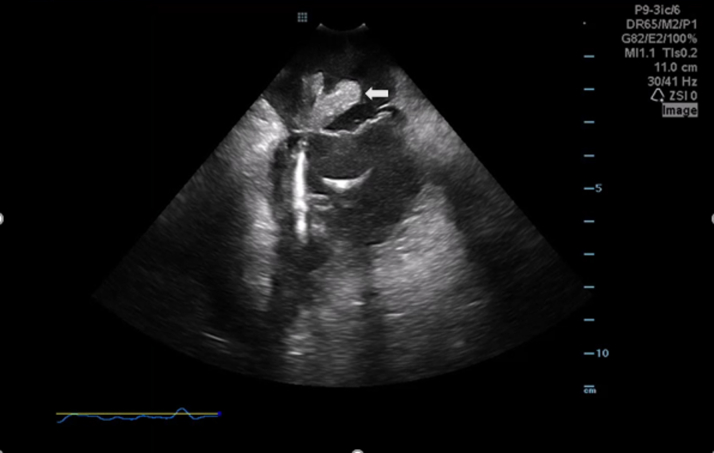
Intracardiac Echocardiography Demonstrating Large, Mobile Echodense Mass Intracardiac echocardiography clearly showed a large mass in the right atrium (arrow), consistent with vegetation.
